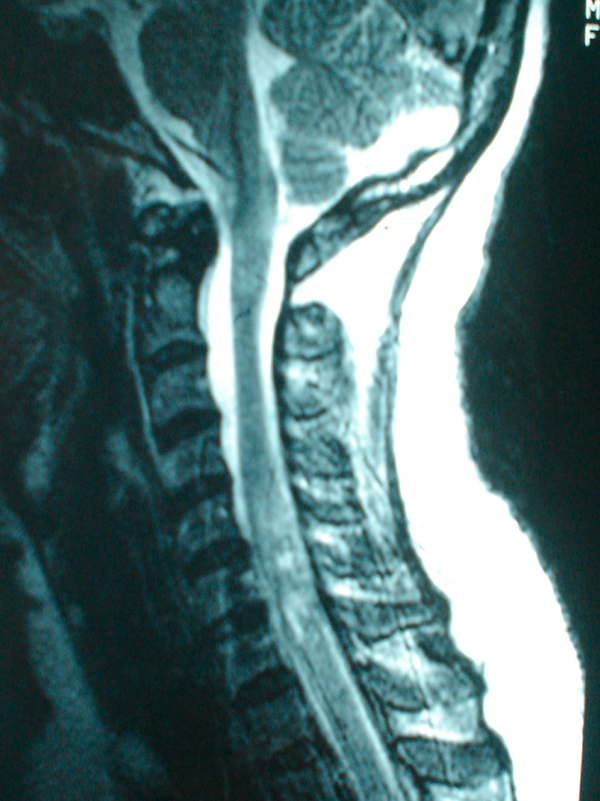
Sagittal T2-weighted image of the cervical spine showing an intramedullary lesion at the C5-C7 spinal vertebrae level.
